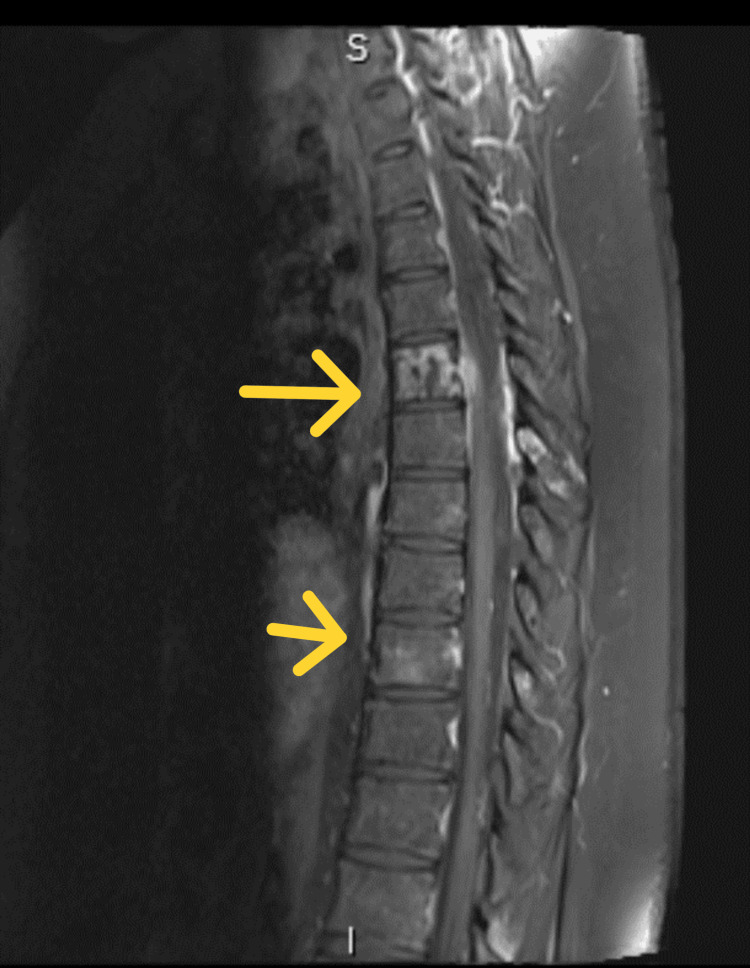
Sagittal MRI of the thoracic spine (T1 with contrast).
